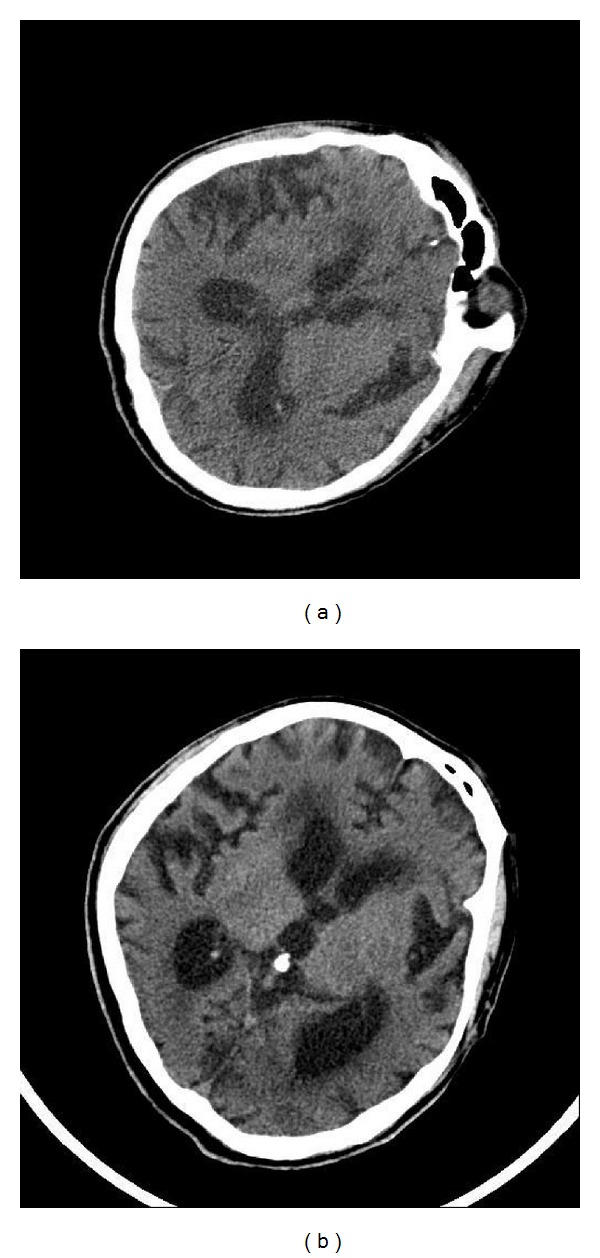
The brain computed tomography (CT) image at onset and admission time. (a) Brain CT image at onset and (b) brain CT image at admission time (3 months after onset).
